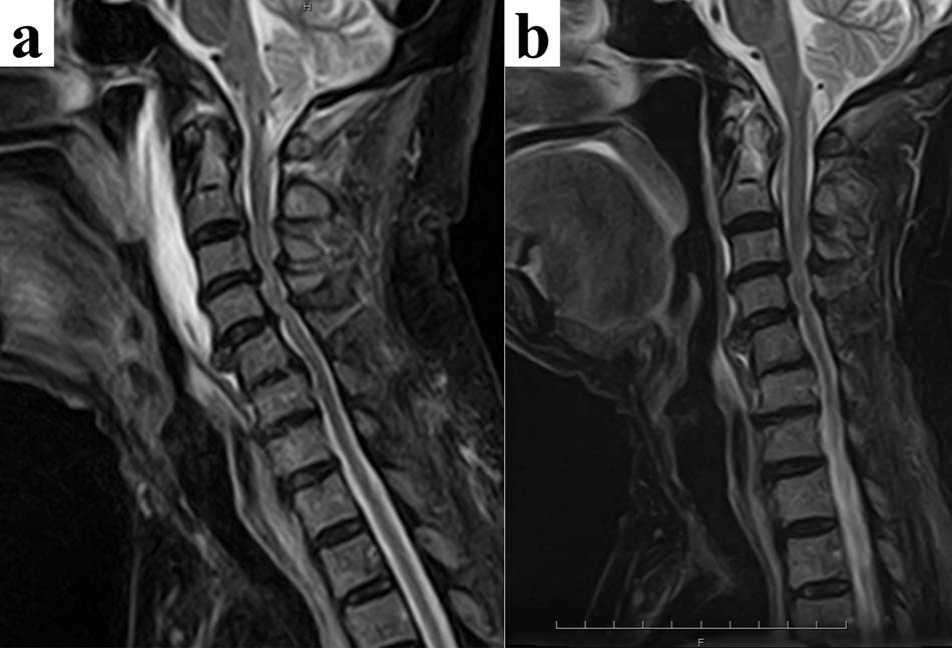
(a) A magnetic resonance image shows a strong signal area in front of the cervical vertebral body which coincides with the longus colli muscle in the STIR sequence. (b) One week after the administration of loxoprofen, the STIR sequence in the magnetic resonance image shows improvement of the swelling and hyperintensity in the longus colli muscle.
